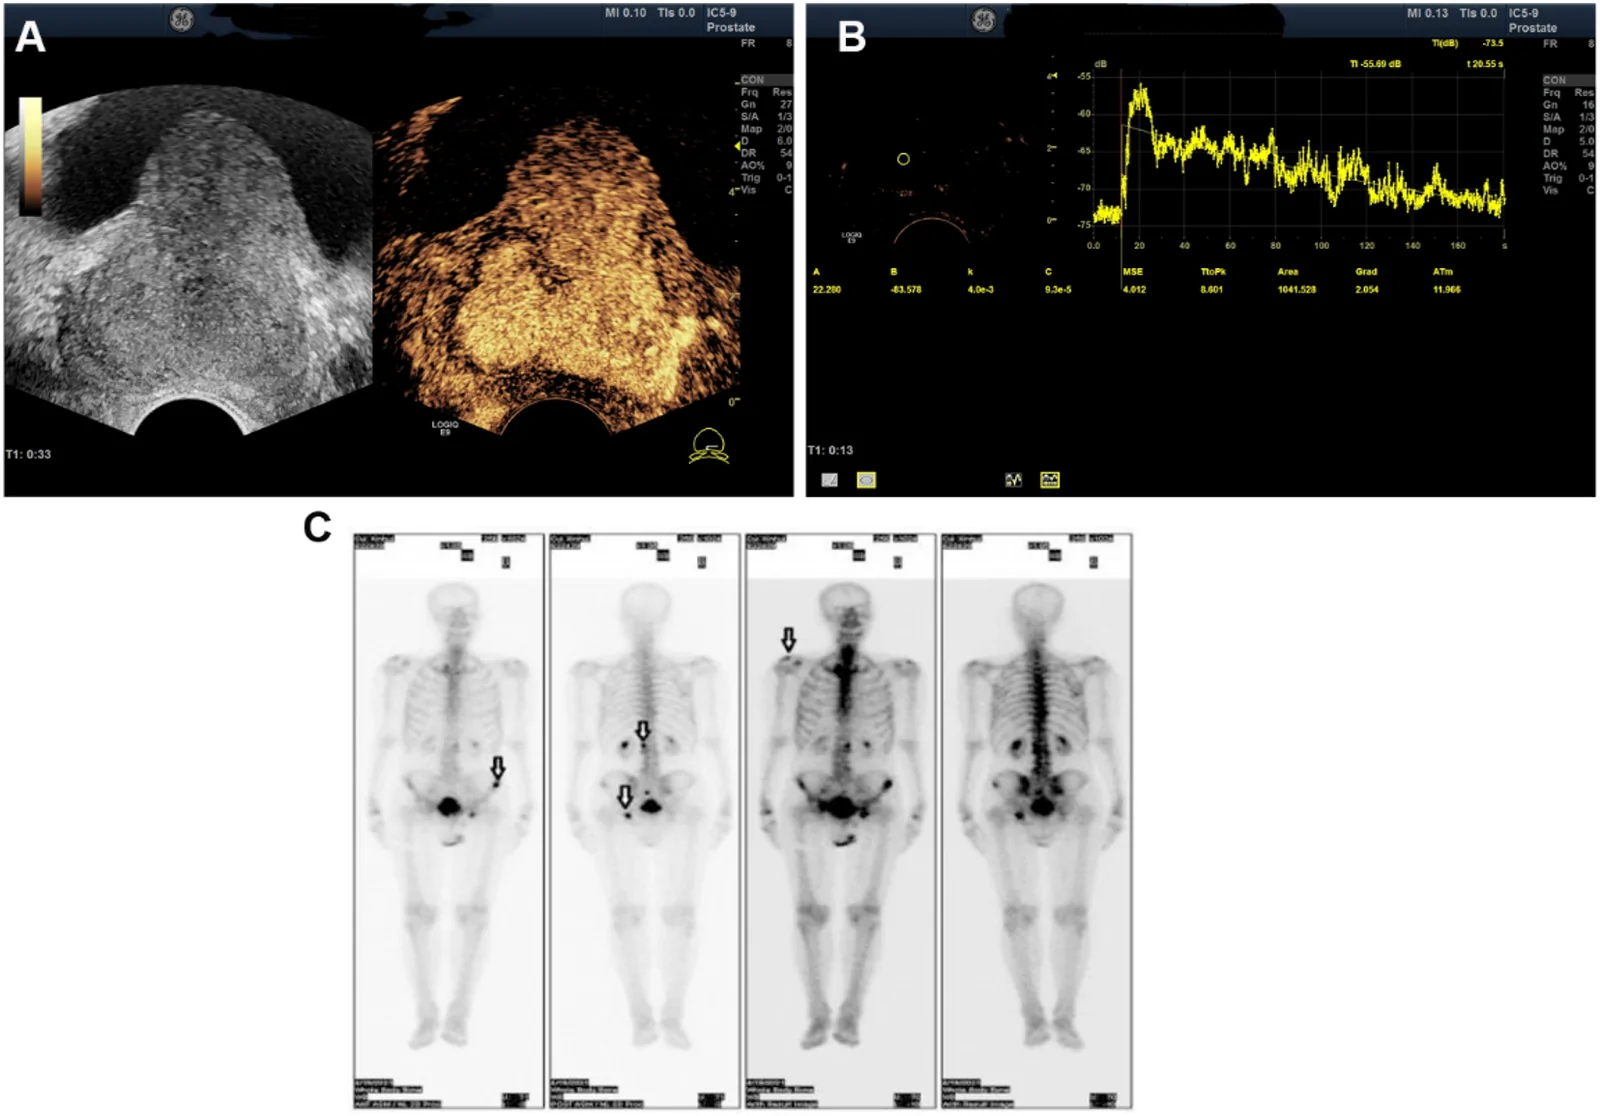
Transrectal ultrasonography, exosome concentrations and whole-body bone scans in patients with BM. (A) Prostagram showing non-uniform hyperenhancement. (B) The TIC curve showed an AUC of 1,408.610 dB s, a PI of 19.53 dB, and a Grad of 2.252 dB/s; (C) whole-body bone scan of a patient with multiple bone metastases involving the second lumbar (L2) and sacral vertebrae.
